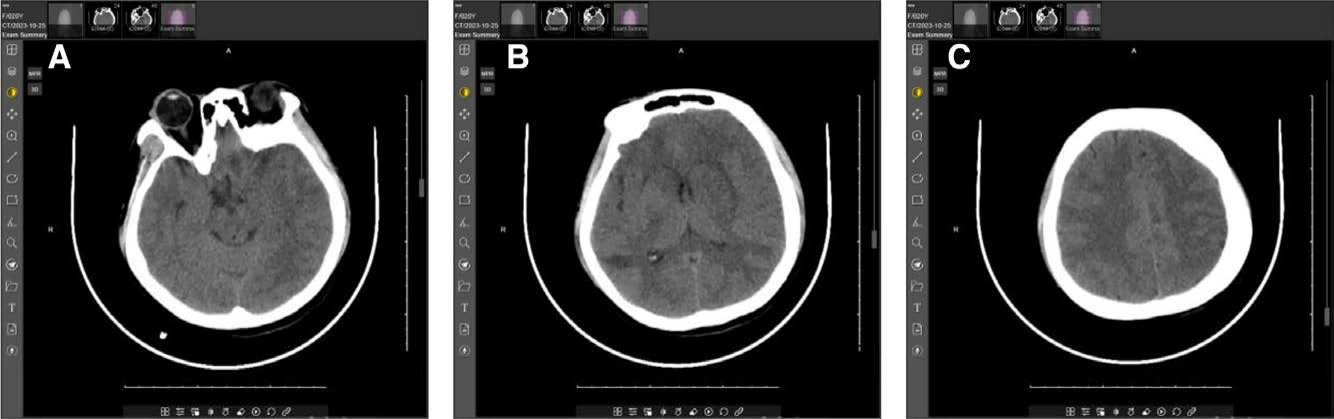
A-1C: October 25, 2023 – CT reveals multiple patchy hypodensities with ill-defined margins in the right frontal and temporal lobes, and bilateral basal ganglia. CT = computed tomography.
